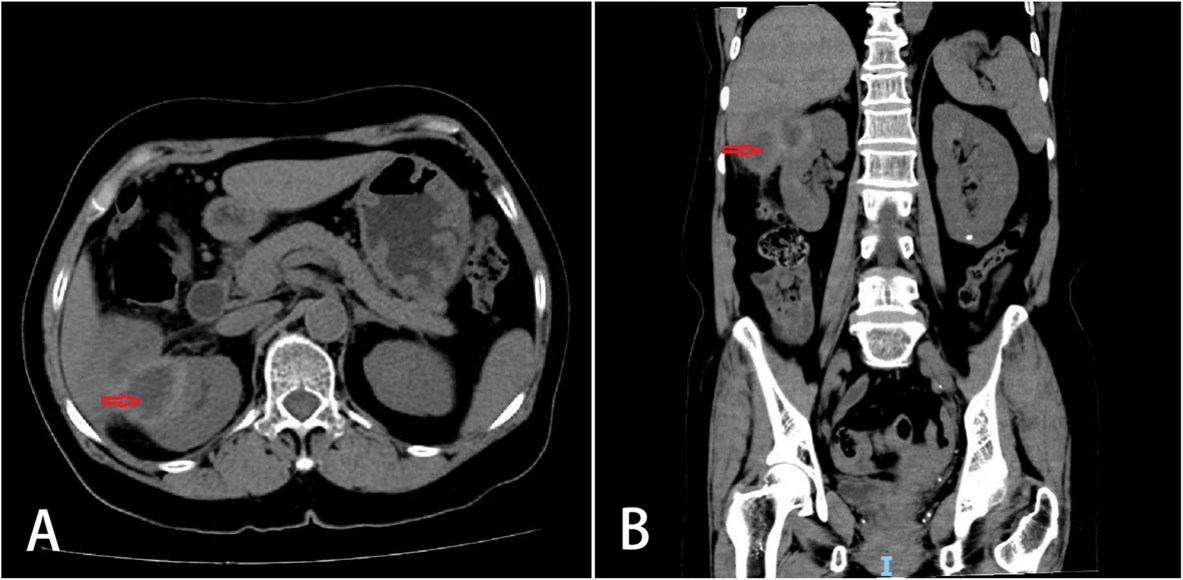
Imaging features of malakoplakia. A Computed tomography coronal view showing a circular low-density focus highlighting the renal profile in the upper part of the right kidney (red arrow). The density is uneven, the surrounding fat space is blurred, and the boundary with the right posterior lobe of the liver and the hepatic curvature of the colon is not clear. B Sagittal computed tomography showing the lesion was gourd-shaped, with low density in the center and slightly higher density in the periphery (red arrow)
